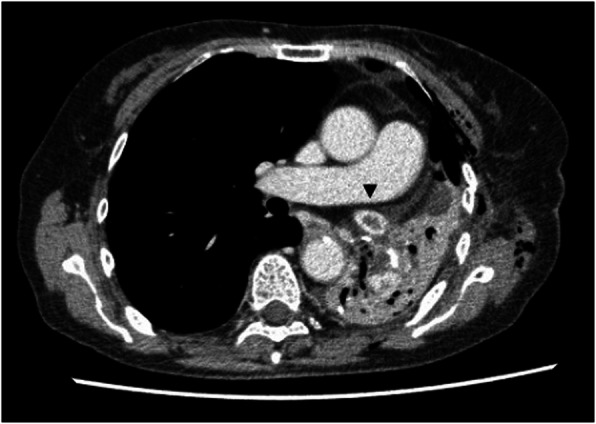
Contrast-enhanced computed tomography showing a thrombus in the left upper pulmonary vein stump in case 3 (arrowhead)
